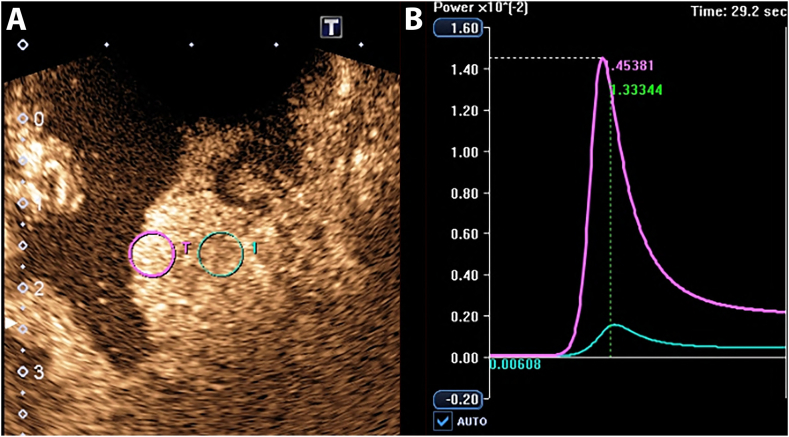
Methods of placing the region of interest (ROI) in the rectal lesion and acquiring the time-intensity curve (TIC) of the contrast-enhanced ultrasound (CEUS) image in a 65-year-old male diagnosed with moderately differentiated rectal adenocarcinoma preoperatively. After total mesorectal resection (TME), pathology showed that 0/17 lymph nodes had no cancer metastasis. (A) CEUS imaging at peak phase. Two ROIs were placed in the rectal cancer lesion: the strongest enhanced ROI (purple) and the weakest enhanced ROI (green). (B) TICs was generated from two ROIs with time on the x-axis and signal intensity on the y-axis. Use the analysis software that comes with the system, and perform fitting calculations to obtain the corresponding quantitative parameters. (For interpretation of the references to color in this figure legend, the reader is referred to the Web version of this article.)
